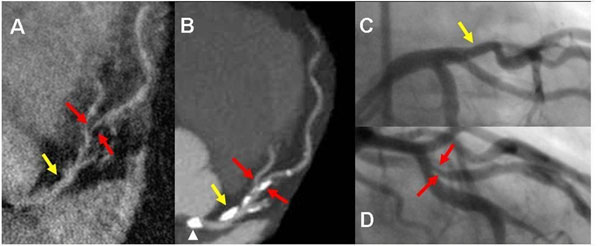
Demonstrates none-significant stenosis in the proximal LAD (yellow arrow) and significant stenosis in mid LAD (red arrows) in a 76 year old male seen both on HRC (A) 320-detectors CT (B), and conventional angiogram (C and D). Despite compromised flow in high grade stenosis, signal is adequate for visualization on the HRC image. A. Multiplanar reformatted image of HRC MRA. B. Maximum intensity projection (MIP) in left anterior view of the LM/LAD. C. and D. Conventional angiogram of LAD in left anterior oblique views. Note calcification (arrow head) in the left cusp partially projecting over the origin of the LM on the MIP due to image plane (B) is not causing severe narrowing as seen on the conventional angiogram (C) or HRC (A).
